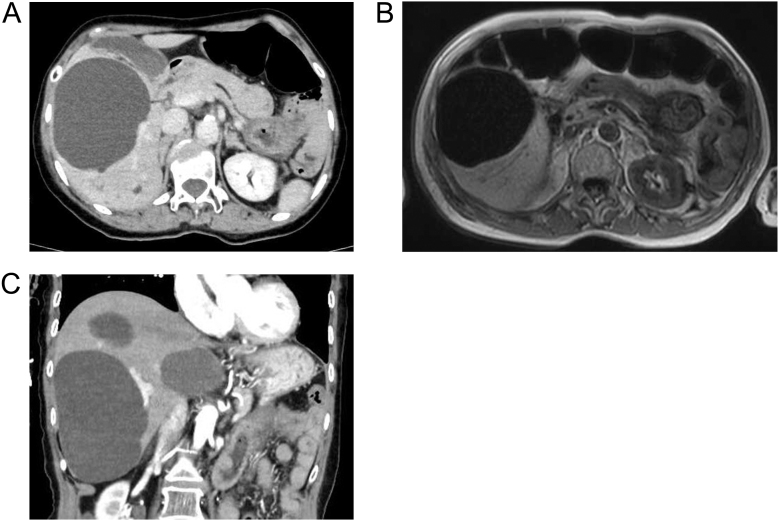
Imaging findings after hospitalization. (A) Dynamic contrast-enhanced CT of the pancreas showed no significant abnormalities. (B) MRI image of the pancreas showed no significant abnormalities. (C) Dynamic contrast-enhanced CT of the liver showed multiple hepatic cysts.
